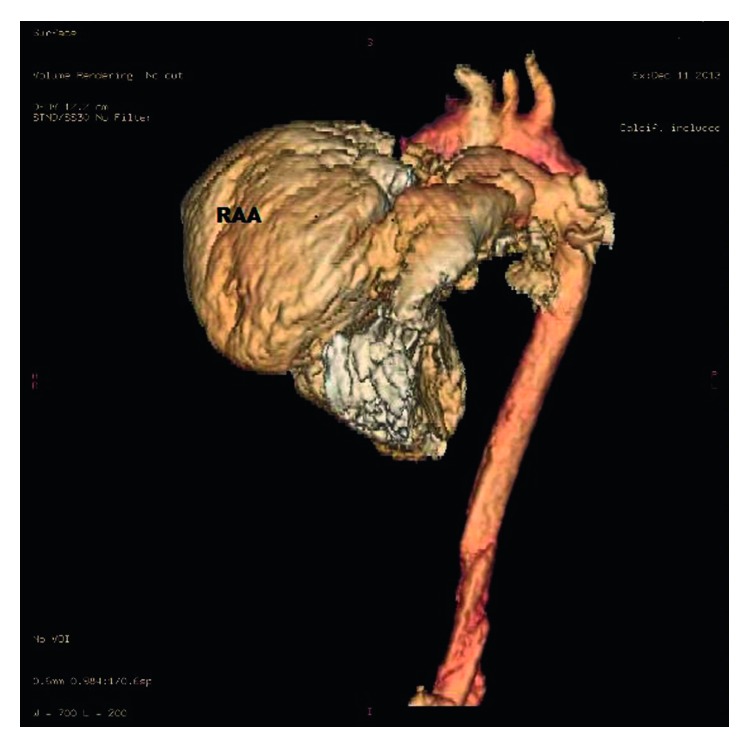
Right atrial aneurysm by three-dimensional CT angiography. RAA: right atrial aneurysm.
